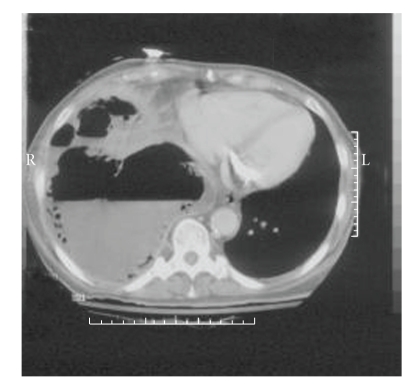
Representative section from the patient's chest CT scan, performed during the second admission. Most of the right chest is taken up by multiple collections of fluid and gas. Note the leftward deviation of the mediastinal structures.
